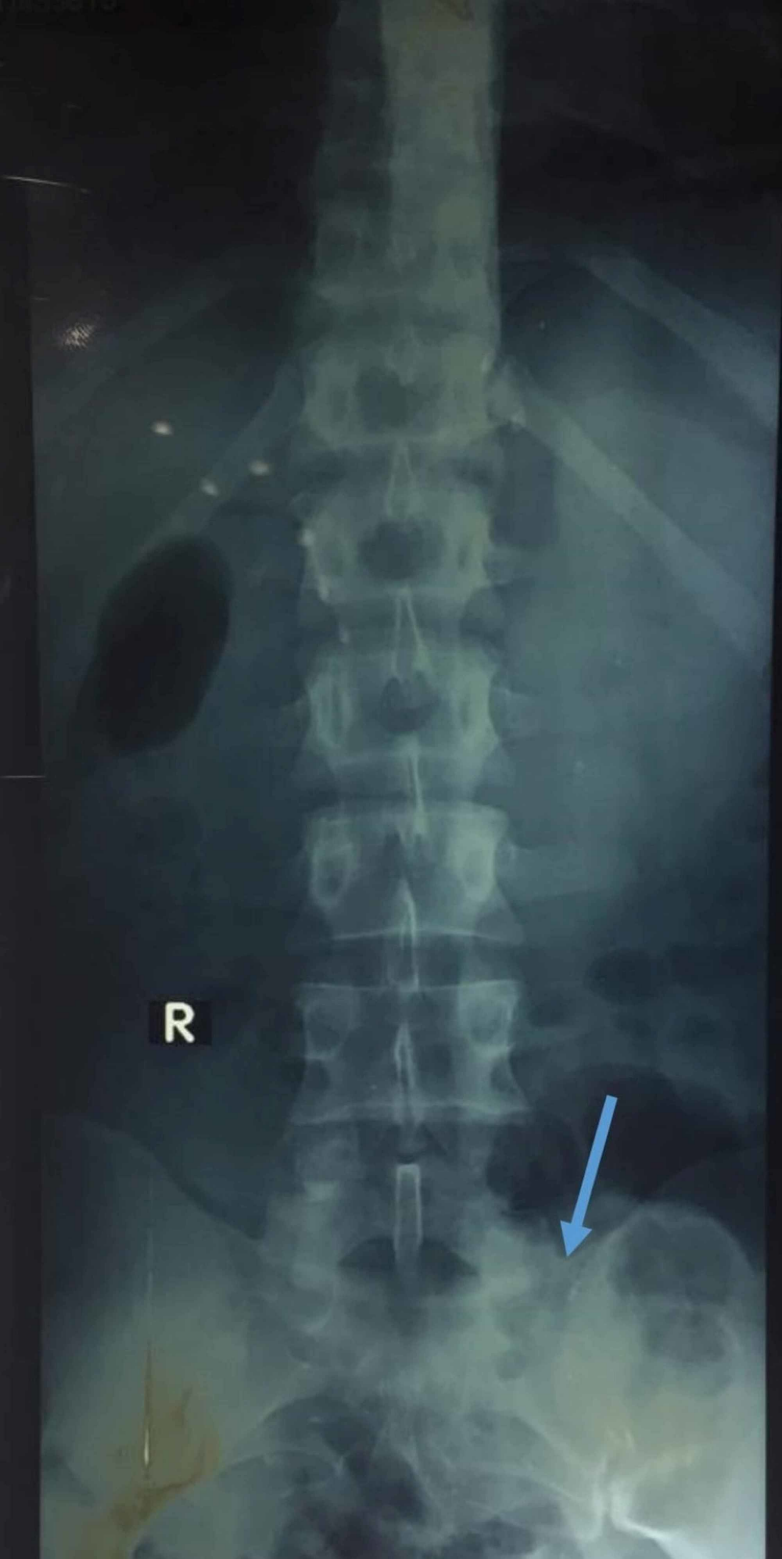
Anteroposterior pelvic radiograph showing sacralization of the lumbar vertebrae (blue arrow)
